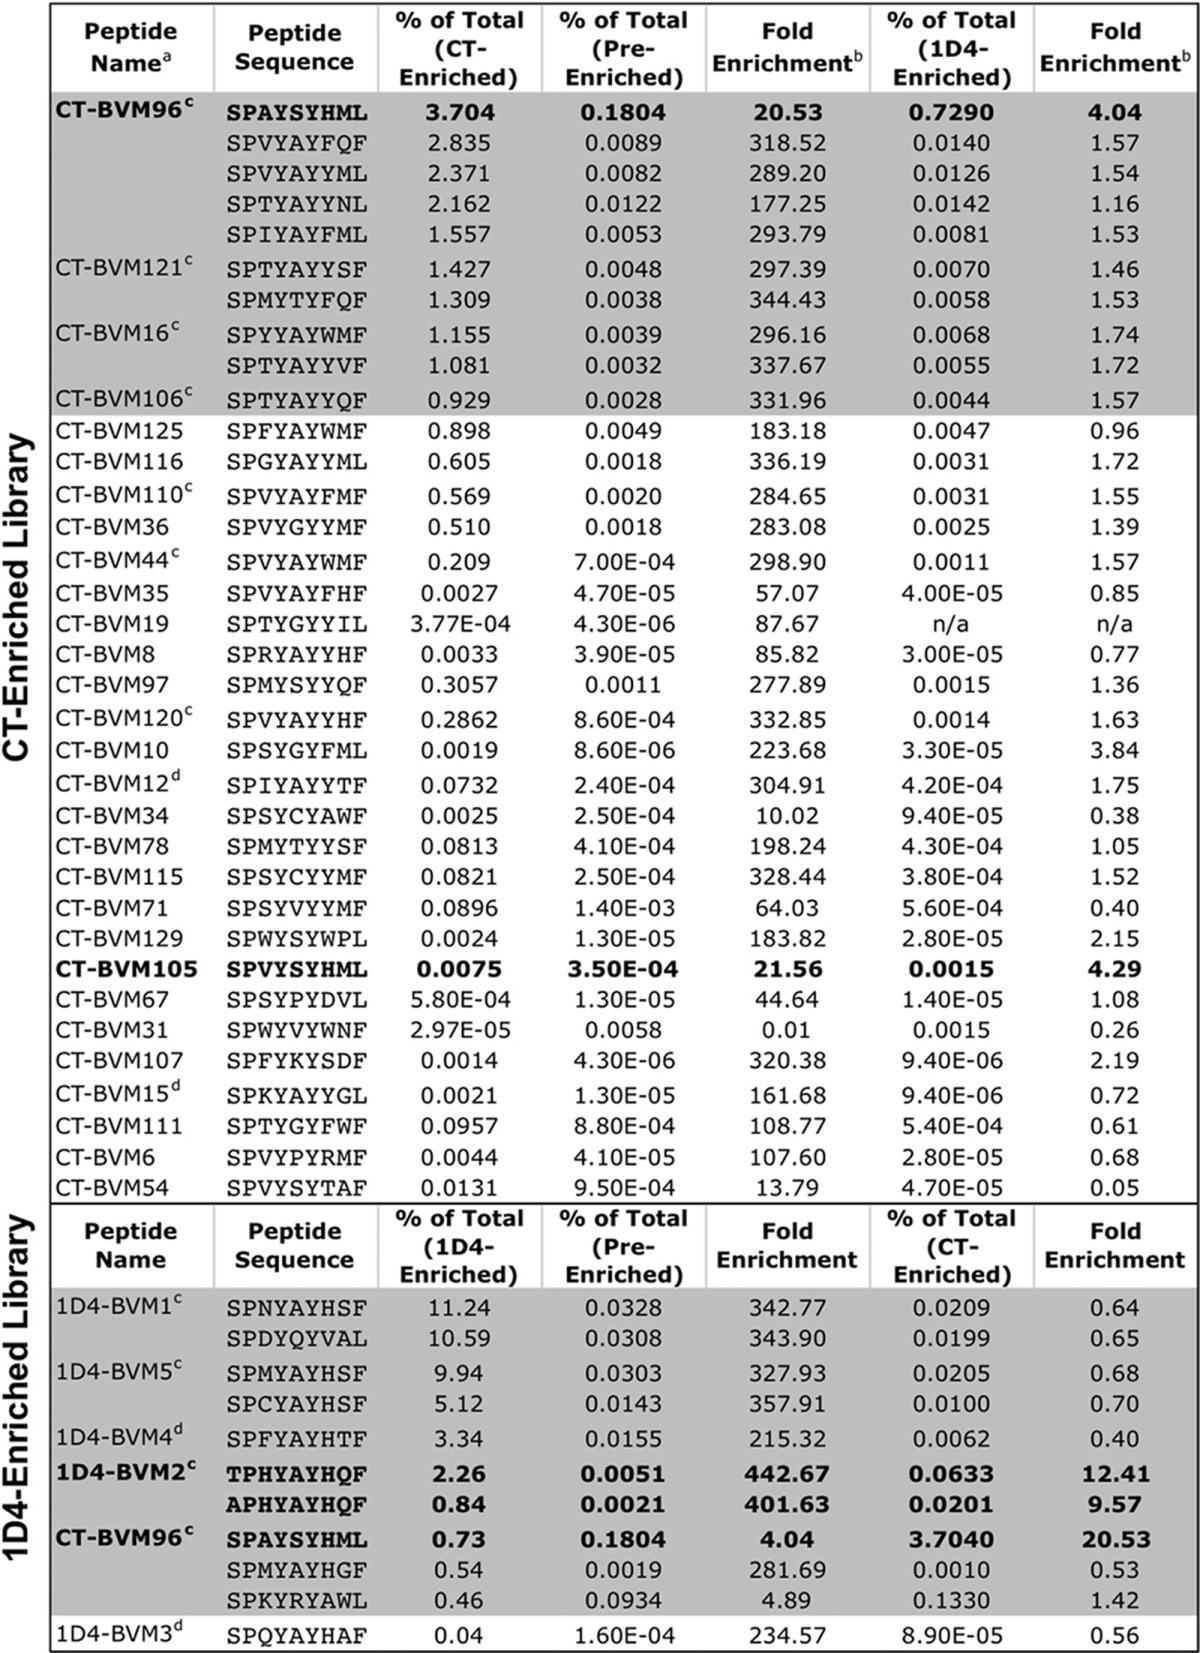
Multiple mimotopes from the BVM library screened by CT and 1D4 TCRs were identified and cloned Gray shading indicates the 10 most frequently identified peptides from each library. Boldface type indicates mimotopes that were enriched >4-fold using both TCRs.
